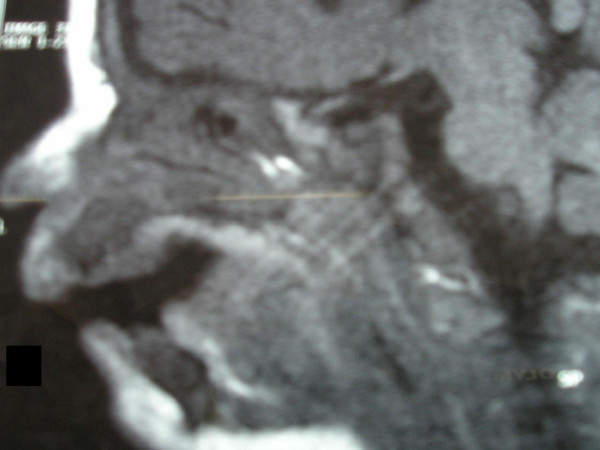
Sagittal MRI imaging, showed markedly hypoplastic tongue.
